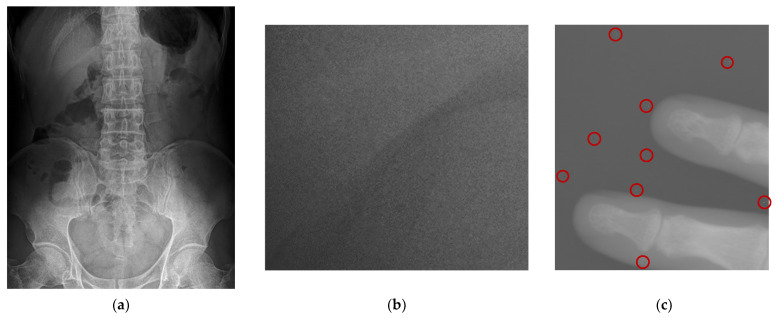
Noise in digital radiography image. (a) Enhanced abdominal image with uniform fine particles (b) Enlarged detail section (c) Raw DR image of a local hand with speckle noise.
